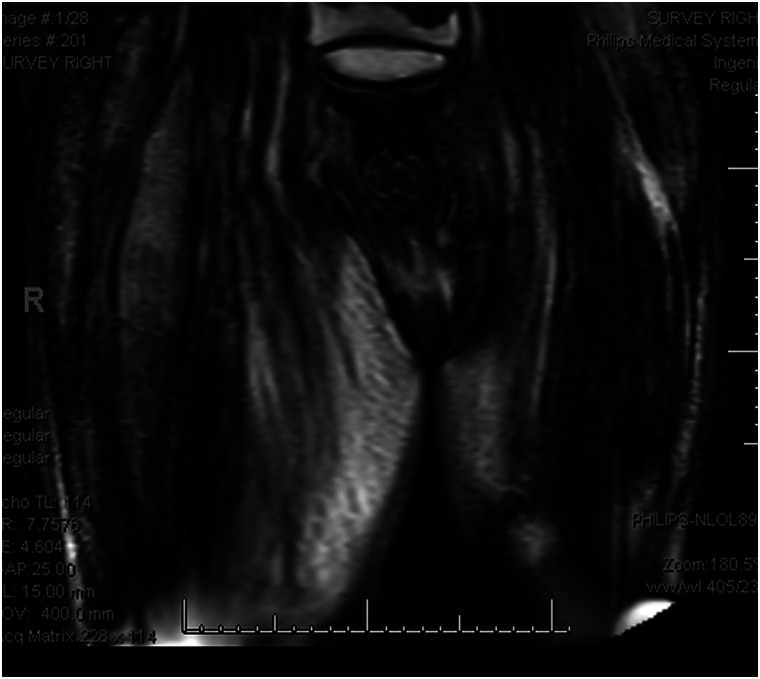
Survey magnetic resonance imaging scan, coronal view of the thighs displaying a size difference of the right and left thigh.
